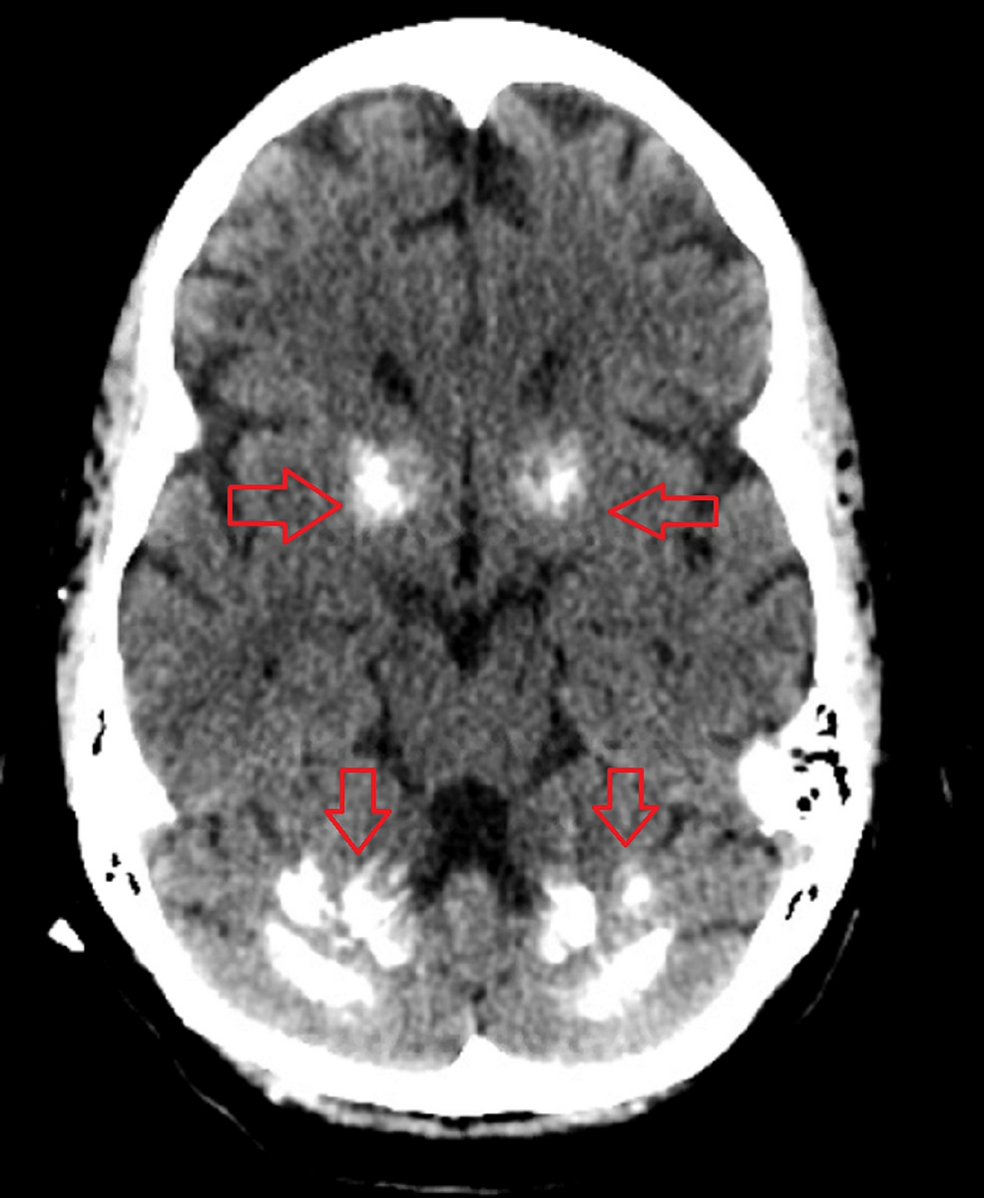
Computed tomography scan of the brain showing bilateral calcification (arrows)
